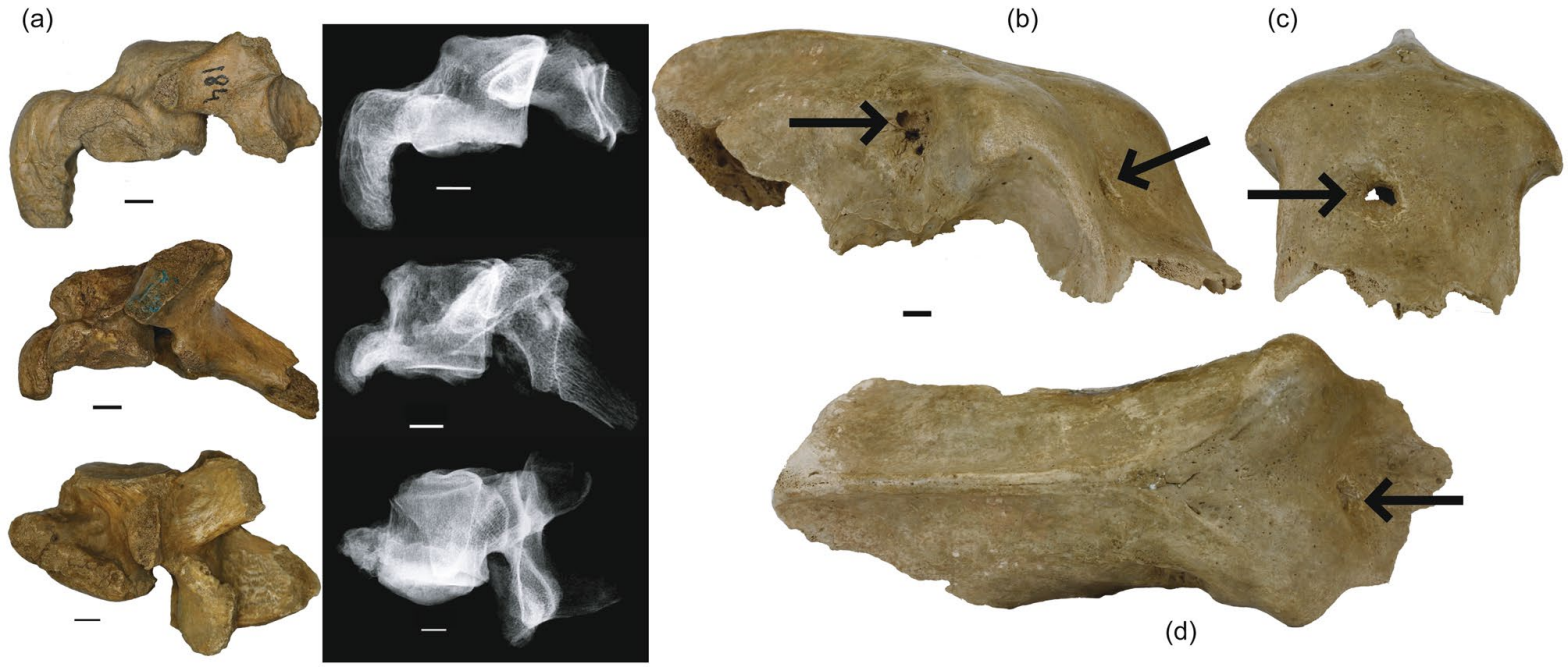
Palaeopathological modifications. (a) Left lateral views and X-ray images, of ossified *ligamenta flava anterior* in three *syndesmophytes*-affected vertebrae. These modifications are observed in various cervical, thoracic, and lumbar vertebrae of Niedźwiedzia Cave’s *Ursus spelaeus ingressus*. (b) Antero-right lateral view of the skull of a young female *Ursus spelaeus ingressus* from Niedźwiedzia Cave. The left arrow indicates an abscess, likely the direct cause of the individual's demise. The right arrow denotes a frontal bone perforation, likely resulting from the penetration of a tooth during an attack by another predator. (c) The same skull as shown in (b), presented in frontal view. The arrow indicates the frontal perforation shown in (b). (d) The same skull as in (b) and (c), displayed in dorsal view. The arrow indicates the same frontal perforation shown in (b) and (c). Scale bar 1 cm.
